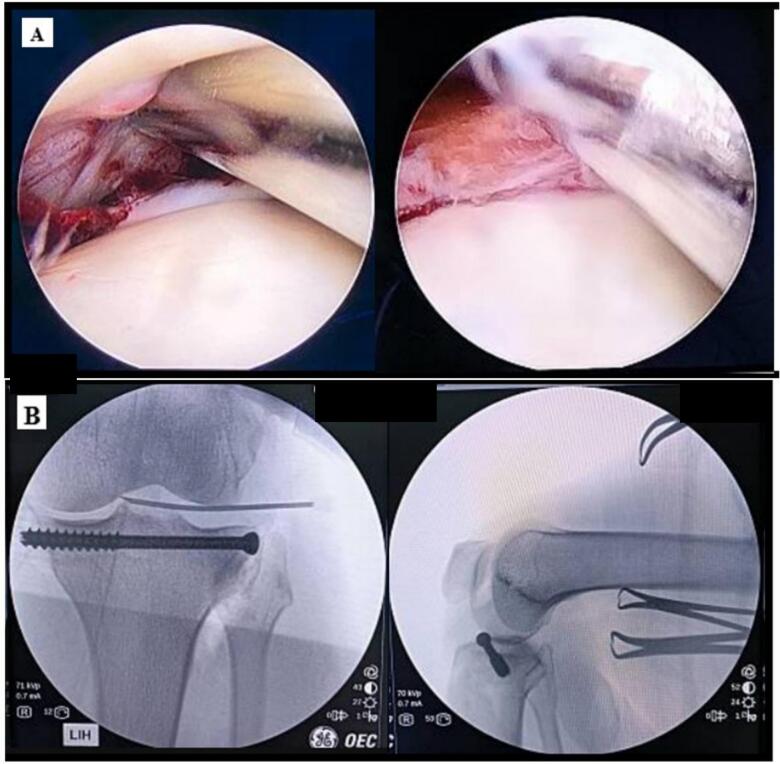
Final Post operative evaluation after surgery. A) Picture from arthrocopy. B) Picture from xray.
